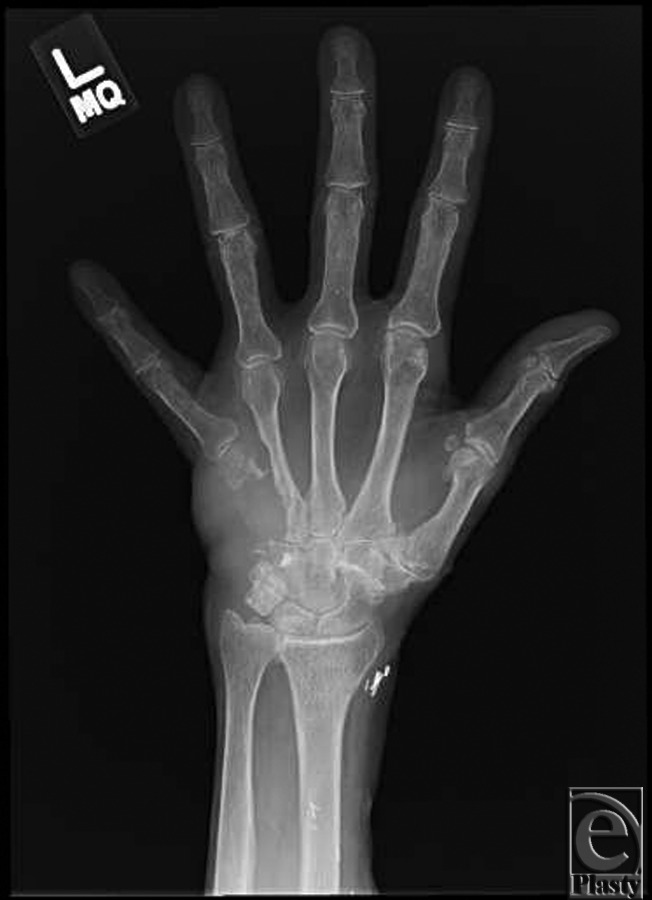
Preoperative radiograph demonstrates a large hypothenar soft tissue mass with osseous destruction of the proximal two thirds of the fifth metacarpal and adjacent cortex of the fourth metacarpal.
